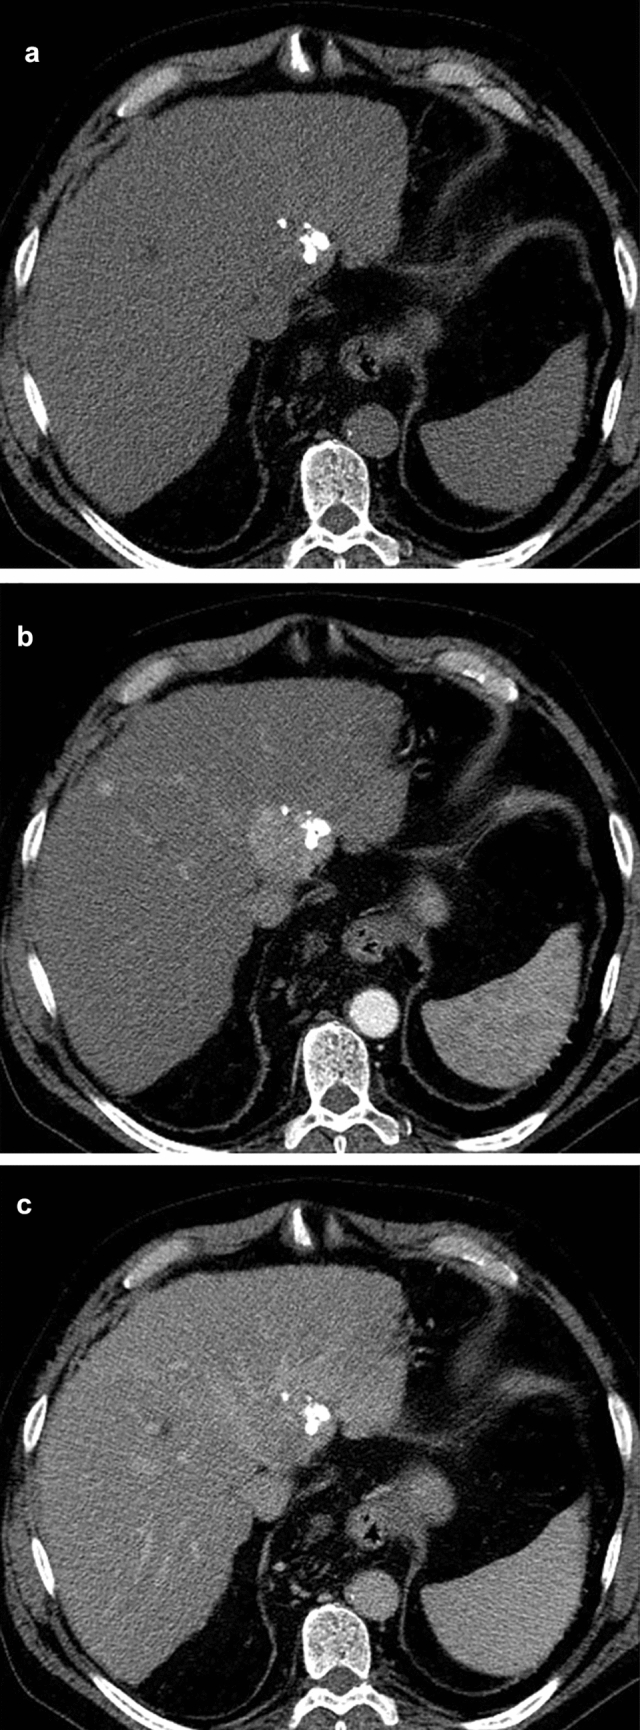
Multiphase CE-CT shows a treated lesion only partially filled with lipiodol. In major part of the lesion mass-like arterial phase hyperenhancement is seen followed by washout appearance in the portal venous phase suggesting post-treatment tumor viability
